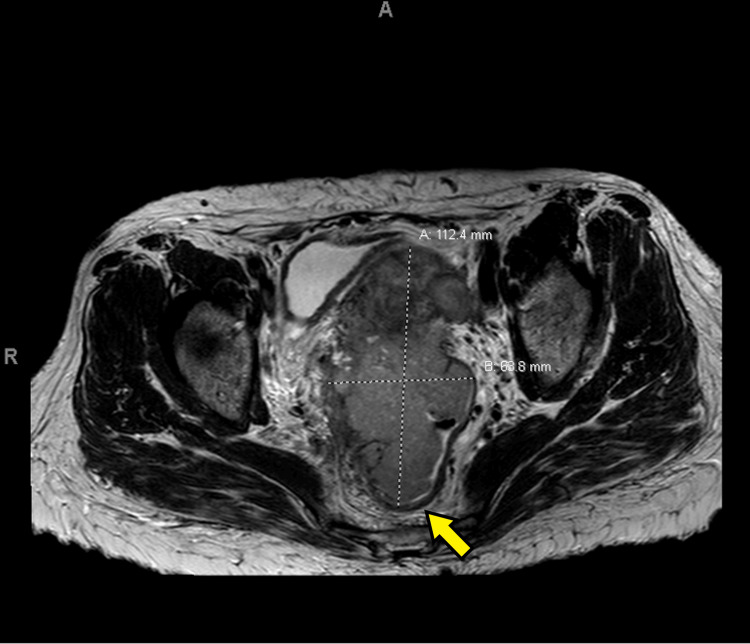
Axial MRI of the cervical lesion at diagnosis The tumor measures approximately 11.2 cm in AP x 6.8 cm in axial dimension. The arrow points at the area of possible rectal involvement.
